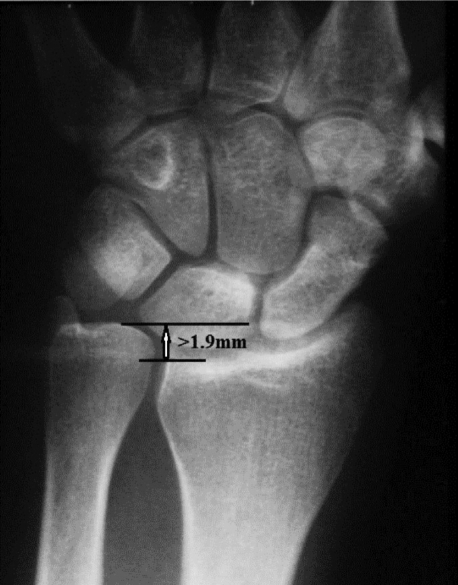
An Essex-Lopresti lesion was diagnosed in this patient's radiographs, which demonstrated greater than 2 mm of shortening of the ipsilateral radius when compared to the contralateral side.
